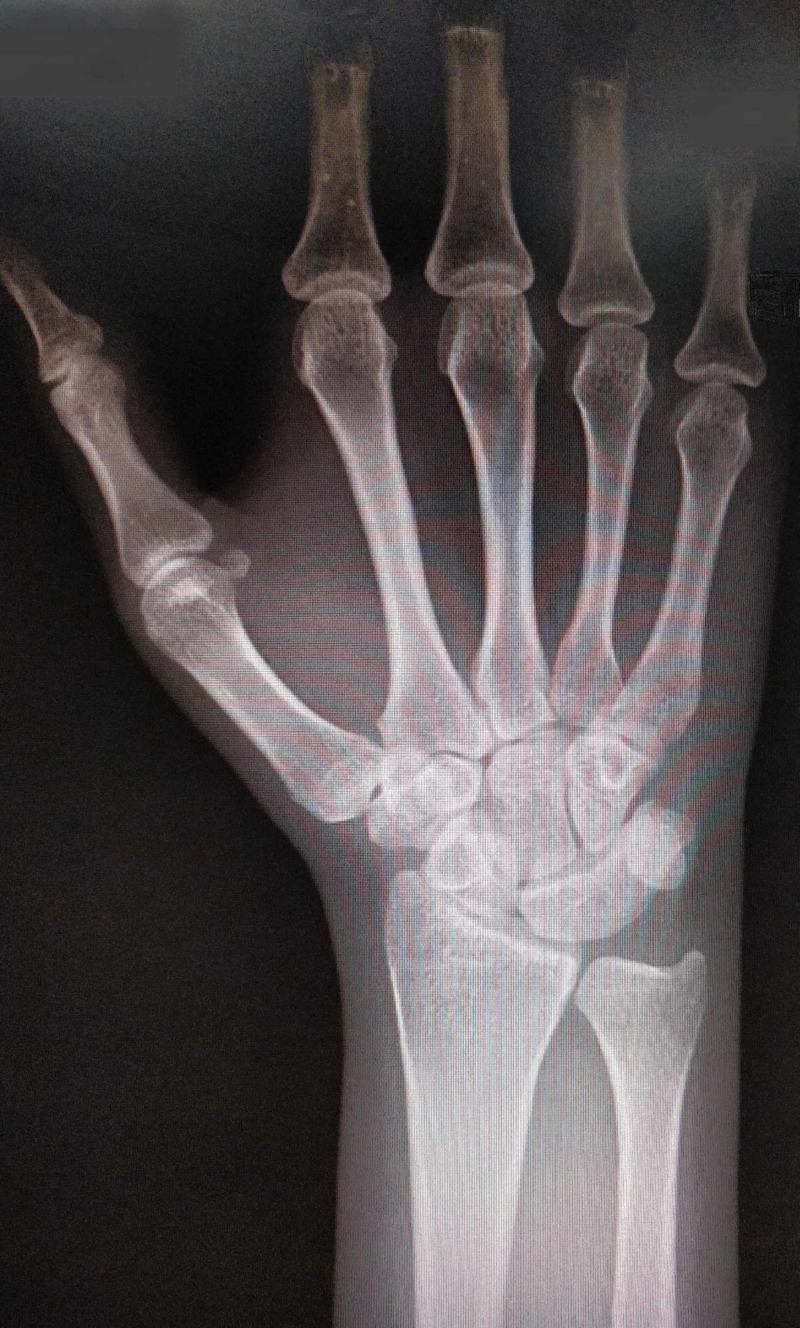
Anteroposterior radiograph
